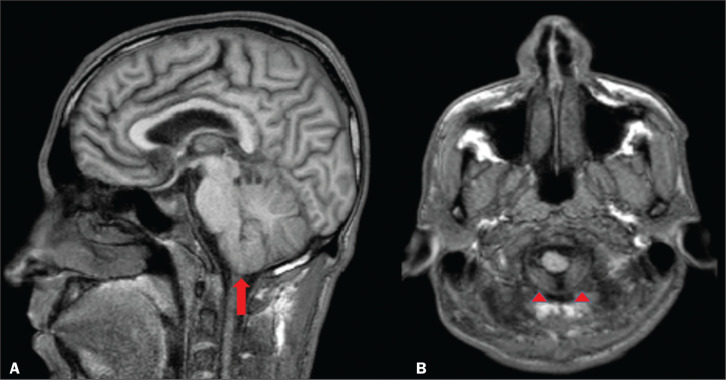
Sagittal and axial T1-weighted MRI scans (A and B, respectively) showing
